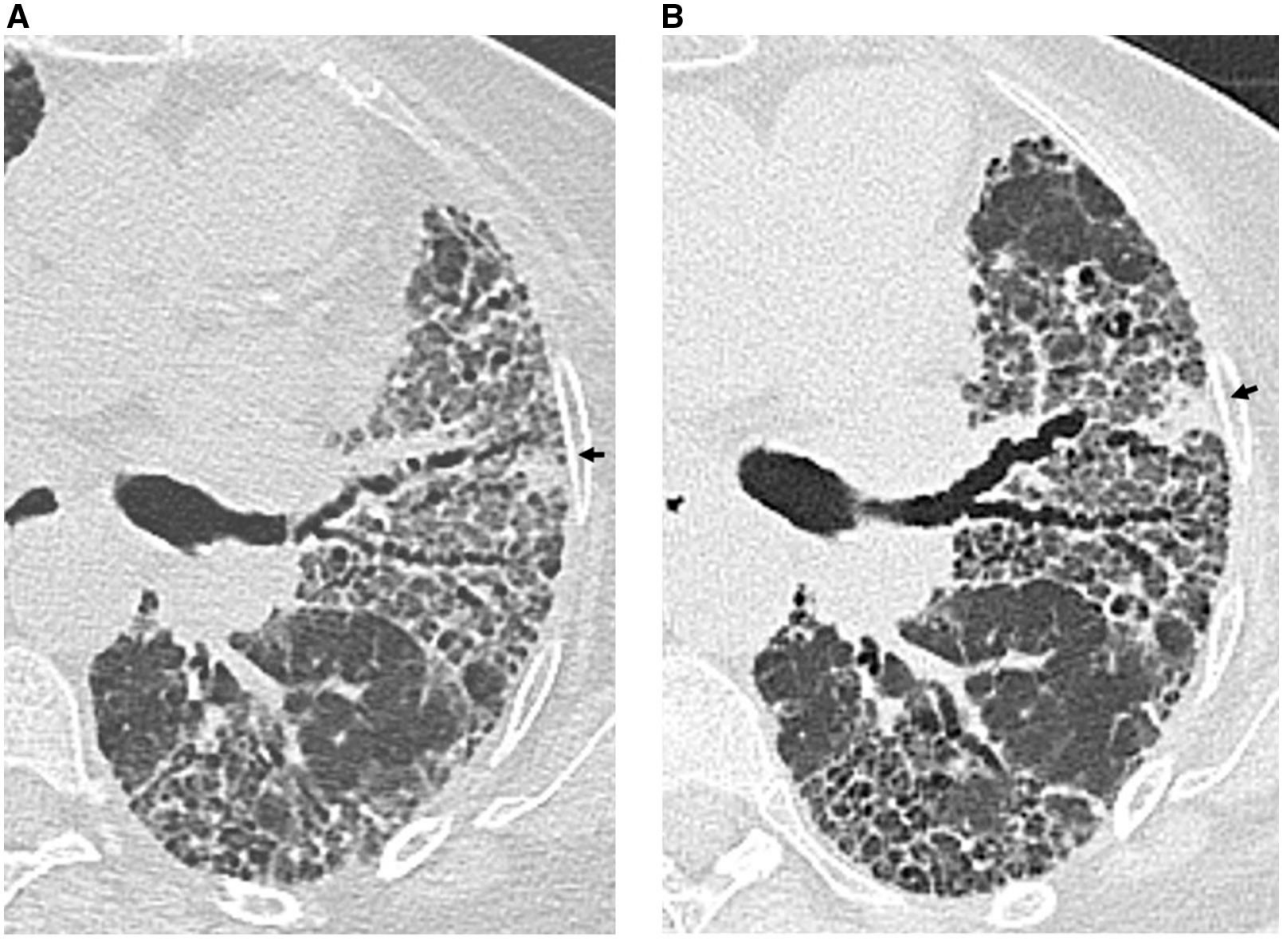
Example of a 72-year-old male patient with fibrotic non-specific interstitial pneumonia (NSIP) scanned 1 year apart. (A) Axial image from an energy-integrating-detector CT (third generation dual-source CT system) (0.75 mm section thickness) obtained at the level of the left upper lobe bronchus, showing CT features of bilateral fibrotic lung disease. (B) Axial PCD-CT image, obtained at the same anatomical level as shown in A 1 year later (0.2 mm collimation, reconstructed with 0.6-mm section thickness, 512 matrix). Compared to A, note the modifications in the patterns of lung involvement in the apical segments of both lower lobes, providing objective clues for disease progression. Note the considerable increase in size of the left upper lobe nodule (*arrow on both images*), highly suspect of malignancy developing in an area of lung fibrosis.
